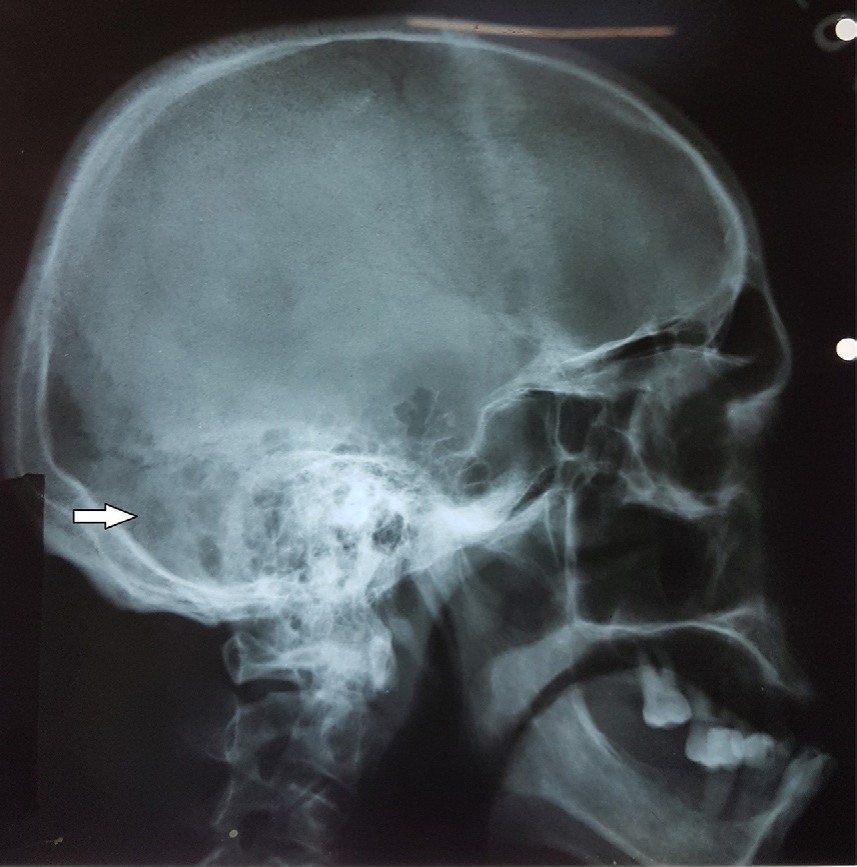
Lateral skull X-ray showing bony erosions (Arrow) suspicious for osteomyelitis.
